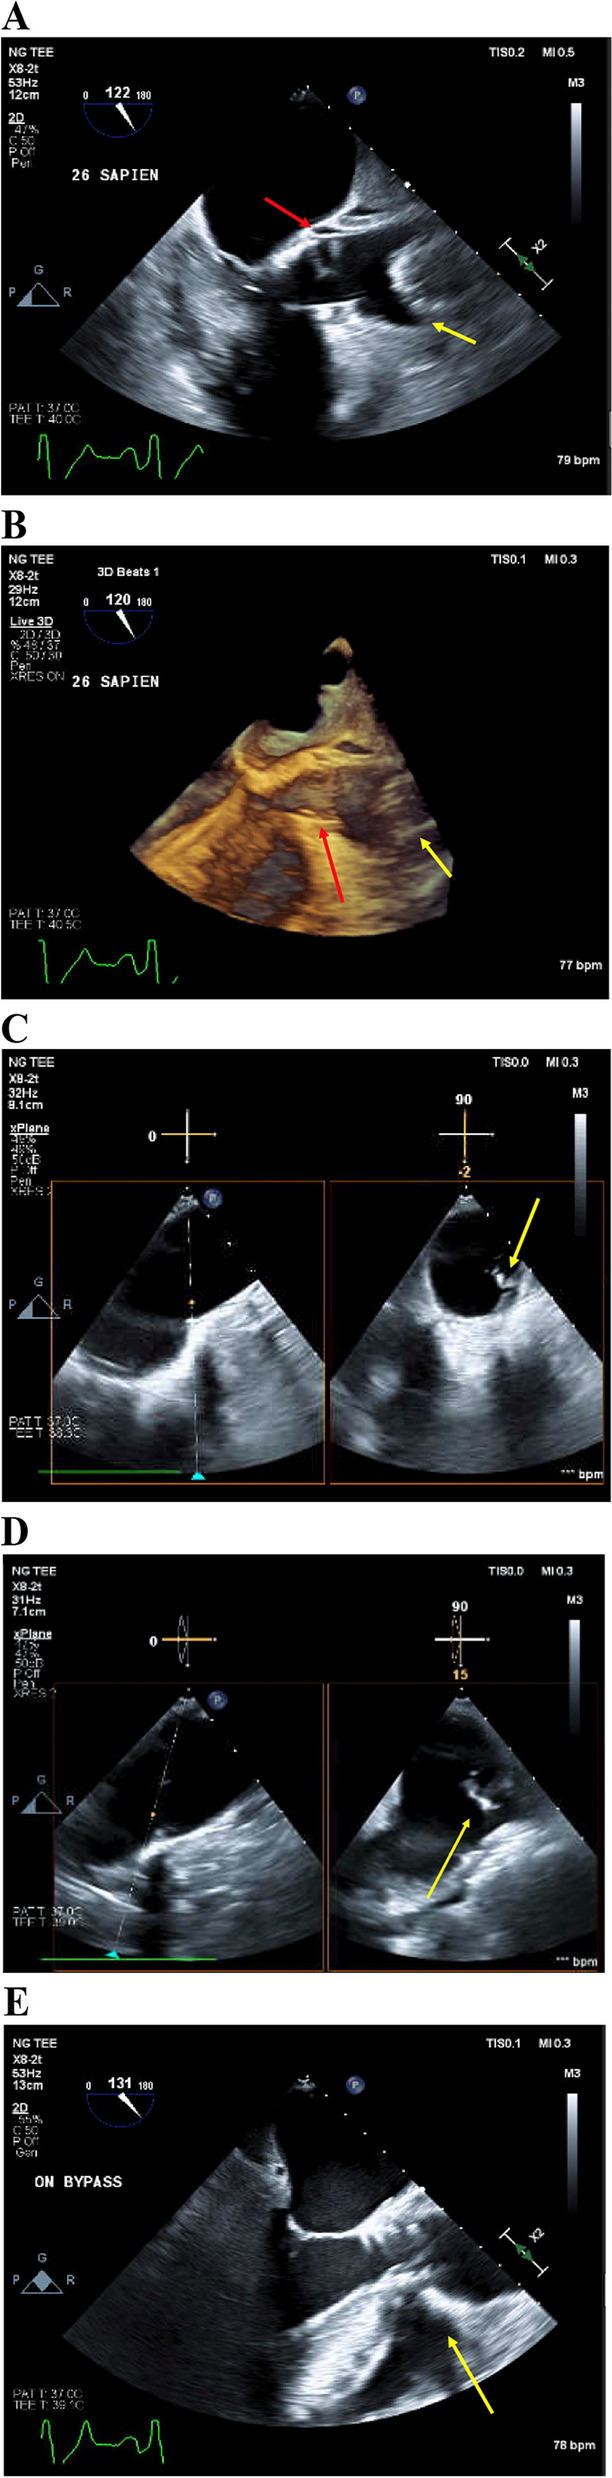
A Transesophageal echocardiography (TEE) mid esophageal long axis 122° 2-dimensional (2D) image showing proper placement of the 26-mm Sapien valve (red arrow) and proximal portion of displaced 29-mm Evolut valve (yellow arrow). The aortic tear located at distal portion of the Evolut valve could not be visualized because the dislodged valve was obstructing the view. B TEE mid esophageal long axis 120 3D imaging showing proper placement of the 26-mm Sapien valve (red arrow) and proximal portion of displaced 29-mm Evolut valve (yellow arrow). C, D TEE short and long axis view without color flow doppler of the aorta showing a 5-mm aortic intimal tear. E TEE mid esophageal at 131° 2D image, showing the 26-mm Sapien valve in proper placement
